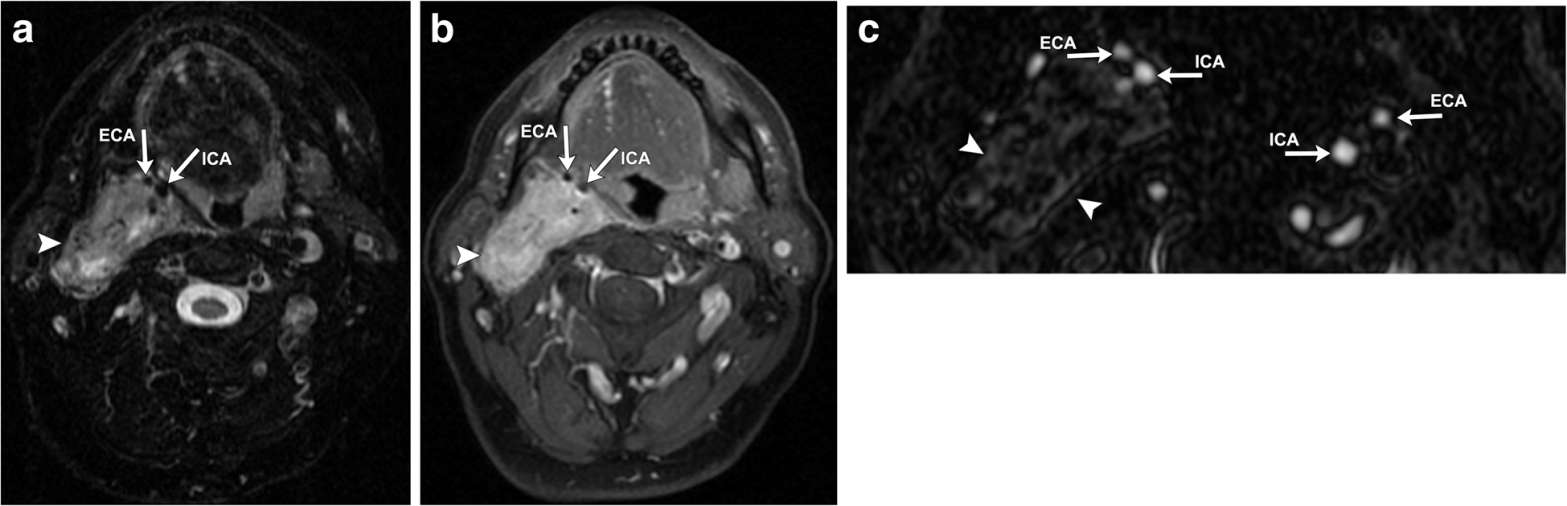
Axial T2 FSE (a) and T1 post-contrast (b) CT images demonstrate a large, well-defined expansile mass centered within the right carotid space (white arrowhead). The lesion is heterogeneously hyperintense on T2 and avidly enhances post-contrast. The flow voids of the right internal and external carotid arteries are displaced together anteromedially (white arrows). Axial 2D time-of-flight MR angiography (c) demonstrates the mass (white arrowheads), as well as clearly delineating its resultant mass effect exerted upon the right ICA and ECA, which are pushed together anteriorly within the neck (white arrows). This is in contrast to the contralateral carotid vessels on the left which are in normal position
